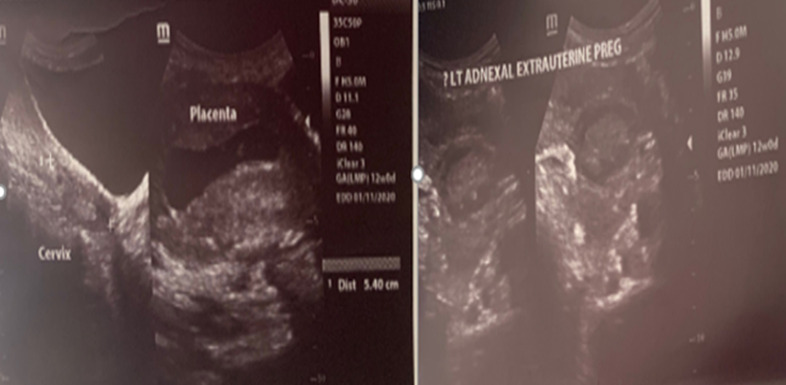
ultrasound scan images showing an intrauterine pregnancy and a left-sided ectopic pregnancy
